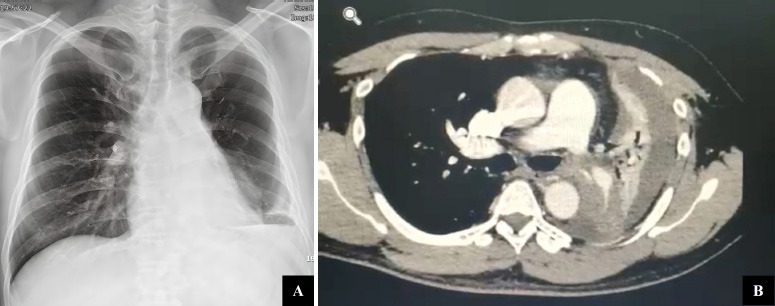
(A) Blunting of the left costophrenic angle on chest X-ray. (B) Chest CT showed an endobronchial tumor in the left main stem bronchus, with lower lobe atelectasis and mild to moderate pleural effusion.
